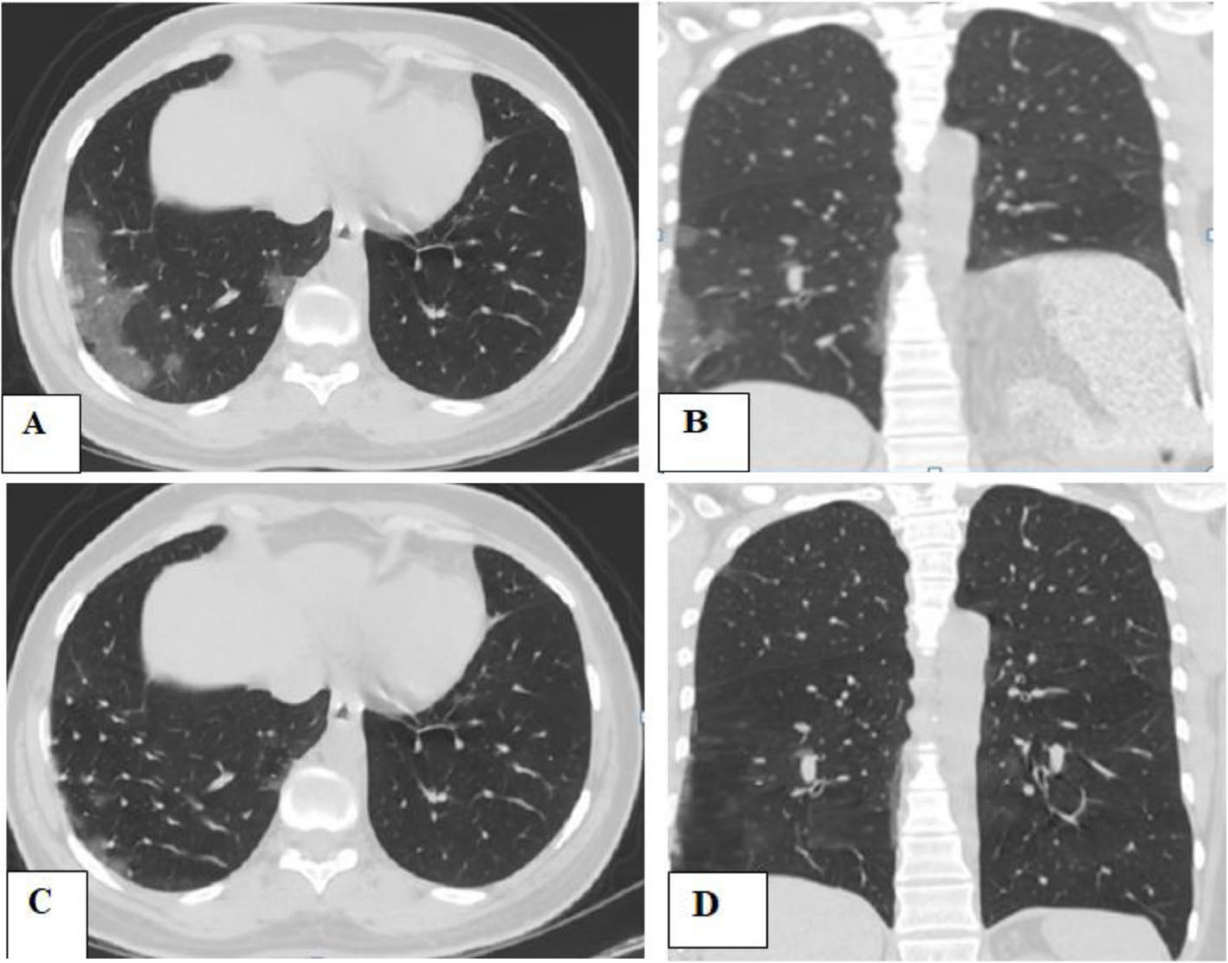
Seventy-eight-year-old male patient, with a history of COVID-19 pneumonia, referred for initial CT evaluation with CT severity score 7. A, B Axial and coronal CT chest imaging respectively showed right sub-pleural ground-glass patches and pulmonary consolidation with interlobar septal thickening. Incidental discovery of left-sided diaphragmatic paralysis. C, D Images after 4 weeks reveals remarkable regression of the right-sided patchy ground glassing and consolidation with resolution of the previous left phrenic paralysis
